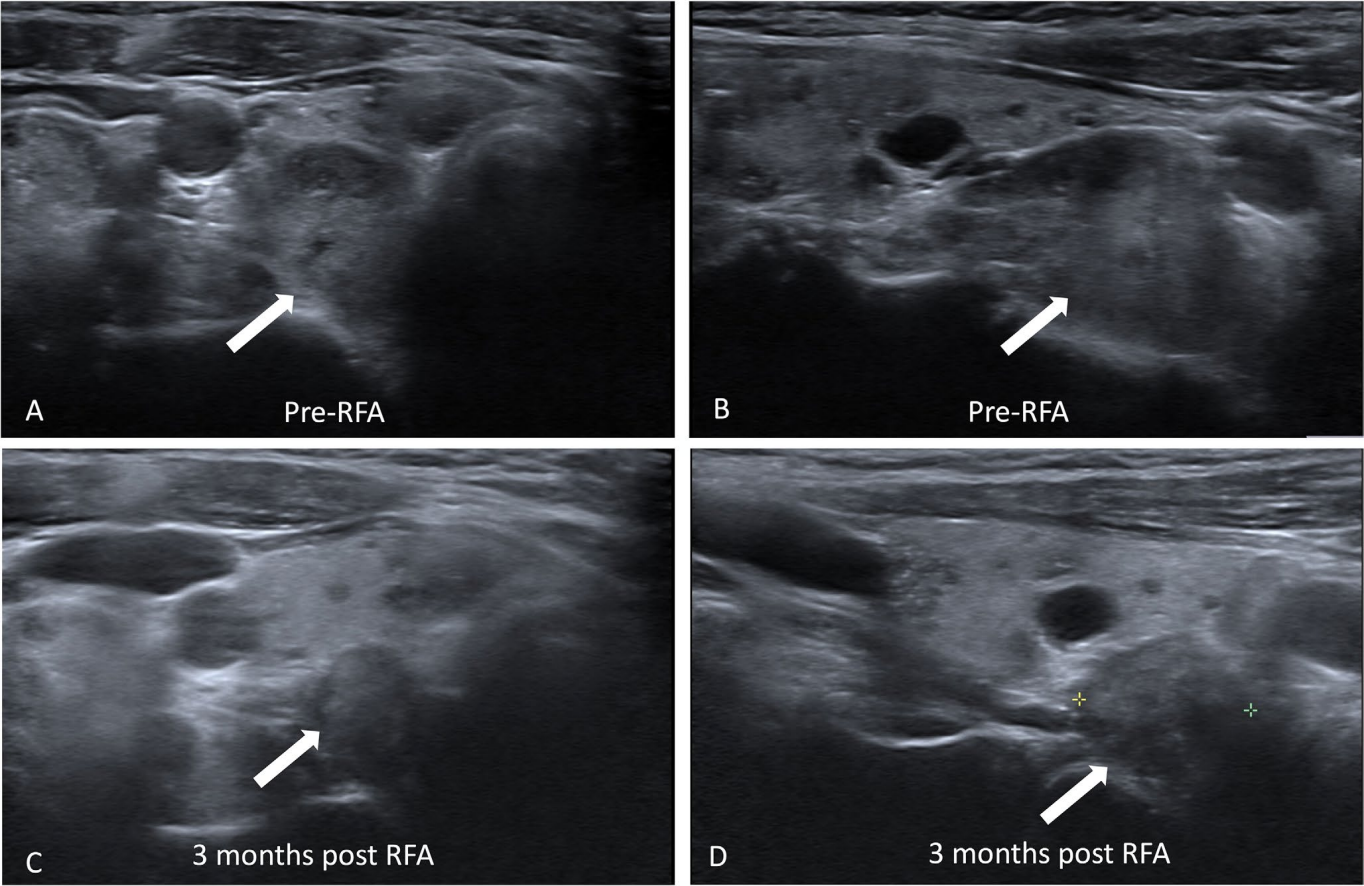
A 66‐year‐old female with primary hyperparathyroidism. (A, B). Pre‐RFA ultrasound examination revealed a right parathyroid adenoma (1.8 x 1.3 x 3.0 cm) (white arrow). (C, D). After RFA, the volume of the nodule (0.9 x 1.1 x 1.4 cm) (white arrow) gradually decreased at the 3‐month follow‐up.
